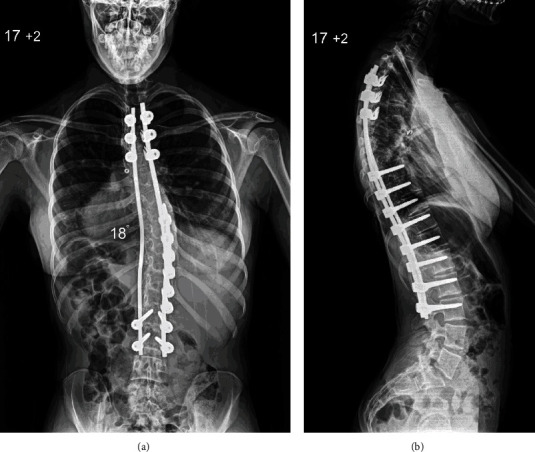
Posteroanterior (a) and lateral (b) radiographs of the spine at skeletal maturity show excellent scoliosis correction and a balanced spine in the coronal and sagittal planes.
